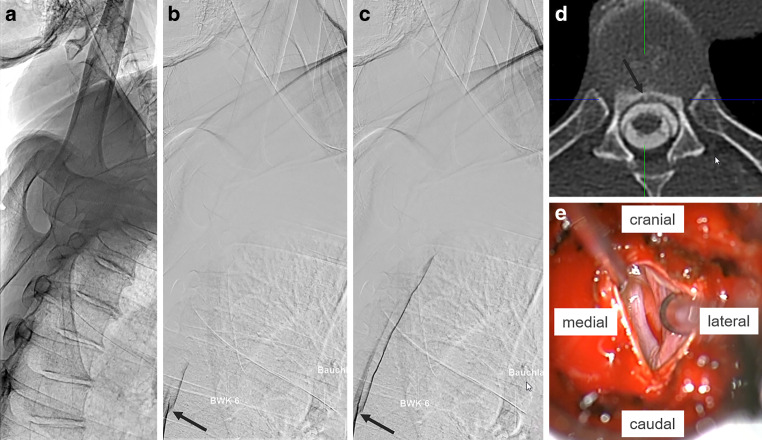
Native lateral X‑ray of patient in prone position with arms overhead in an angiographic suite and a combination of body and table tilting of 20° head down. Left shoulder (humeral head) is elevated over the level of the spinal canal (a). Digital subtraction myelography (*DSM*) in the same position. Incoming contrast agent enters the ventral epidural space at the level of tear at Th 6/7 (*arrow* in b). As the DSM progresses, contrast runs faster toward the head outside the intrathecal space than inside, indicating a large leak (c). Axial spinal CT post myelography with a small bony spur (*arrow*) at the level Th 6/7. The ventral epidural space is filled with contrast agent (d). Intraoperative view from dorsal with a large tear of about 5 mm in the ventral dura in craniocaudal orientation (e)
